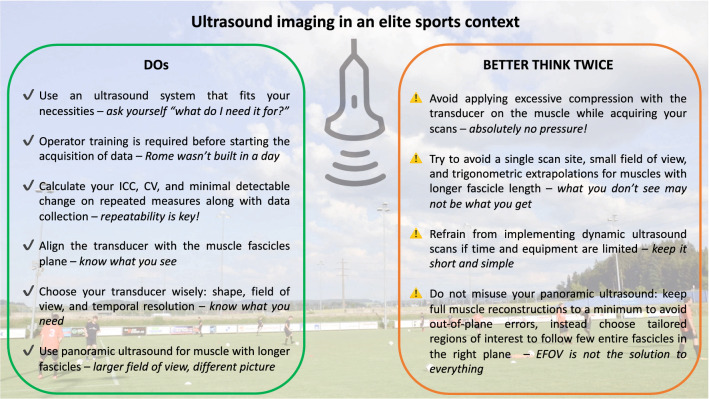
Panel of practical recommendations for the implementation of ultrasound-based imaging in the elite sports context. *ICC* intraclass correlation coefficient, *CV* coefficient of variation, *EFOV* extended-field-of-view ultrasound technique
